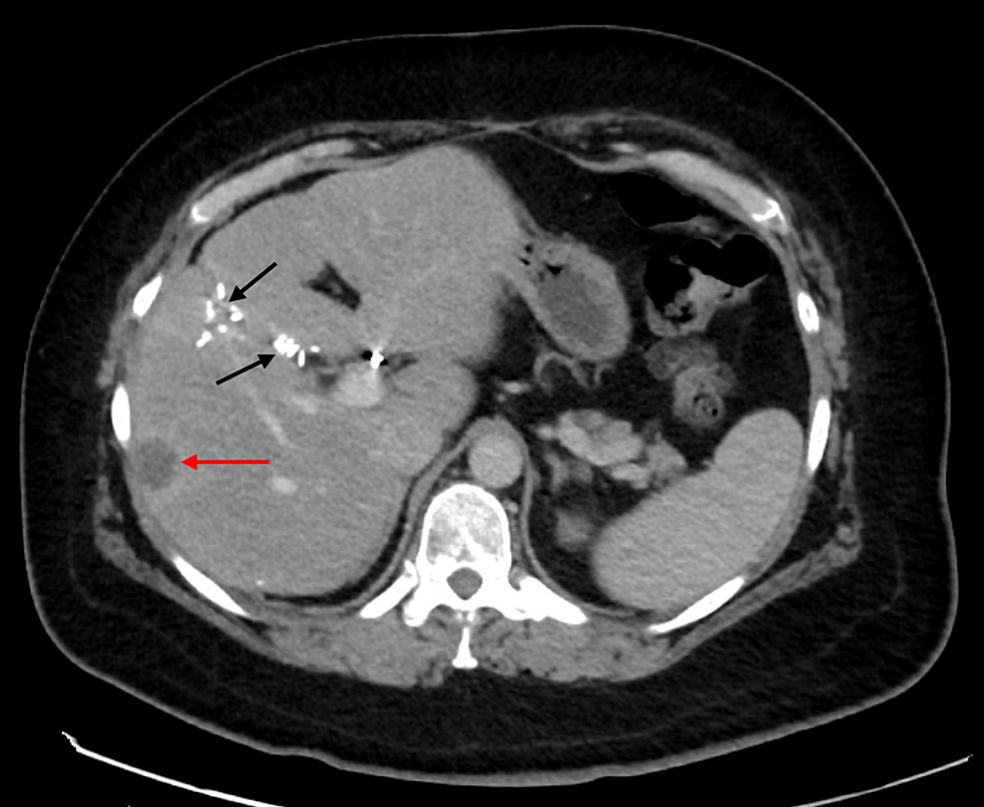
CT abdomen/pelvis in the portal venous phase Scan showing dominant liver metastasis of ~20 mm (red arrow). Surgical clips are seen in situ (black arrows) due to previous surgical resection of liver metastases.
